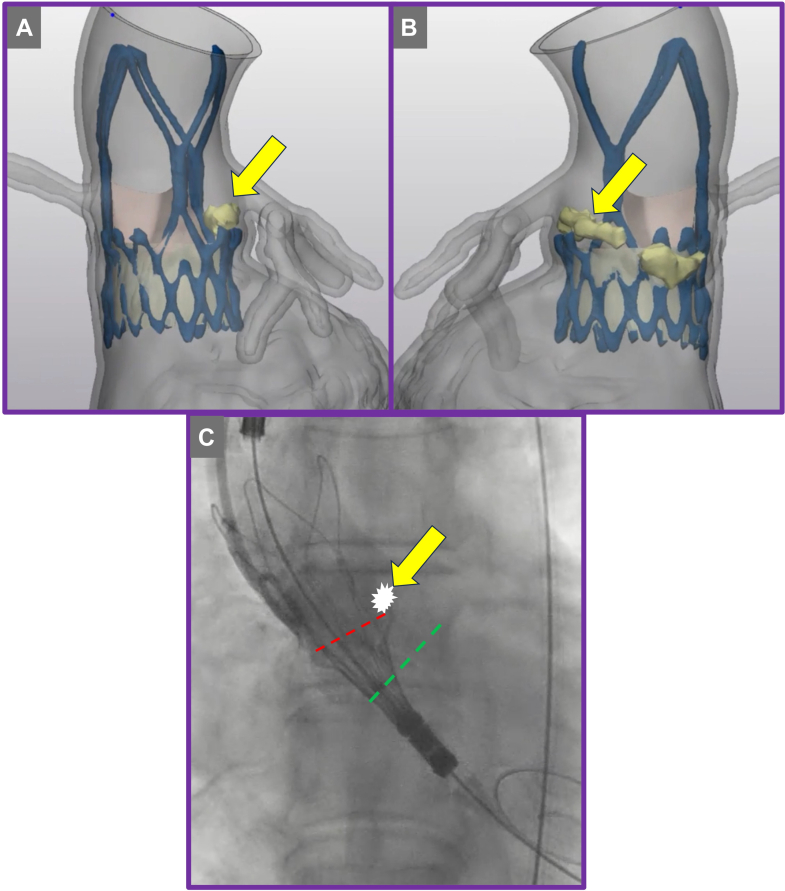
Redo Transcatheter Aortic Valve Replacement Computed Tomography Analysis (A and B) Calcification above the upper crown of the index transcatheter aortic valve replacement prosthesis (yellow arrow). (C) Annular plane (green dashed line) and misaligned deployment plane (red dashed line).
